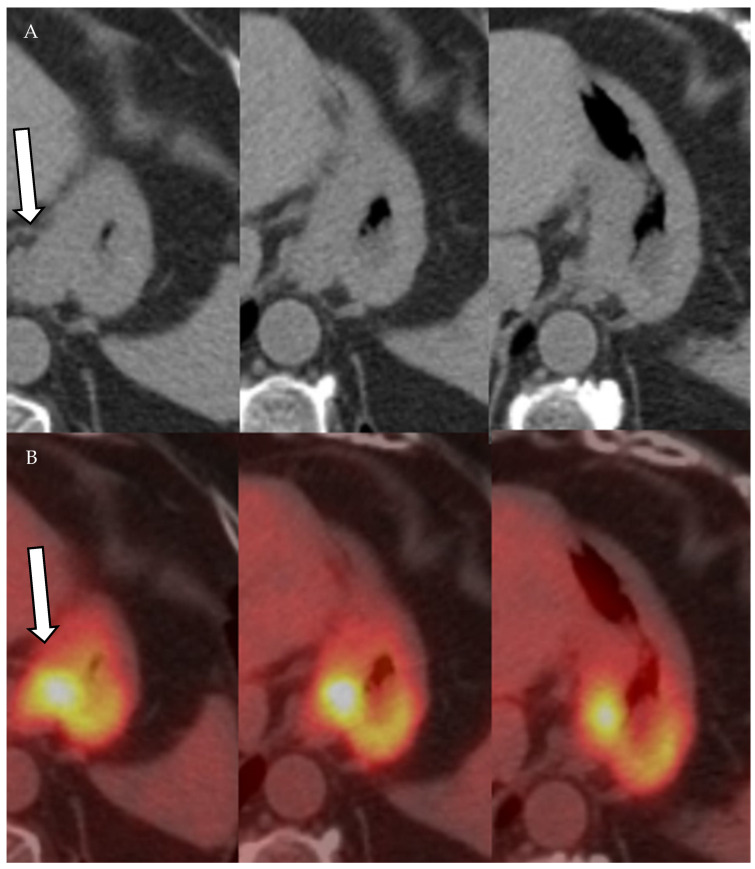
A 61-year-old female patient with history of gastric cancer. PET/CT scan demonstrates diffuse thickening of the gastro-esophageal region as well as the gastric cardia (arrow) (A) consistent with gastric carcinoma, which is FDG-avid (arrow) with maximum SUV = 11.6 (B). There is no focal abnormal metabolic activity to suggest distant metastatic spread or regional lymphadenopathy.
